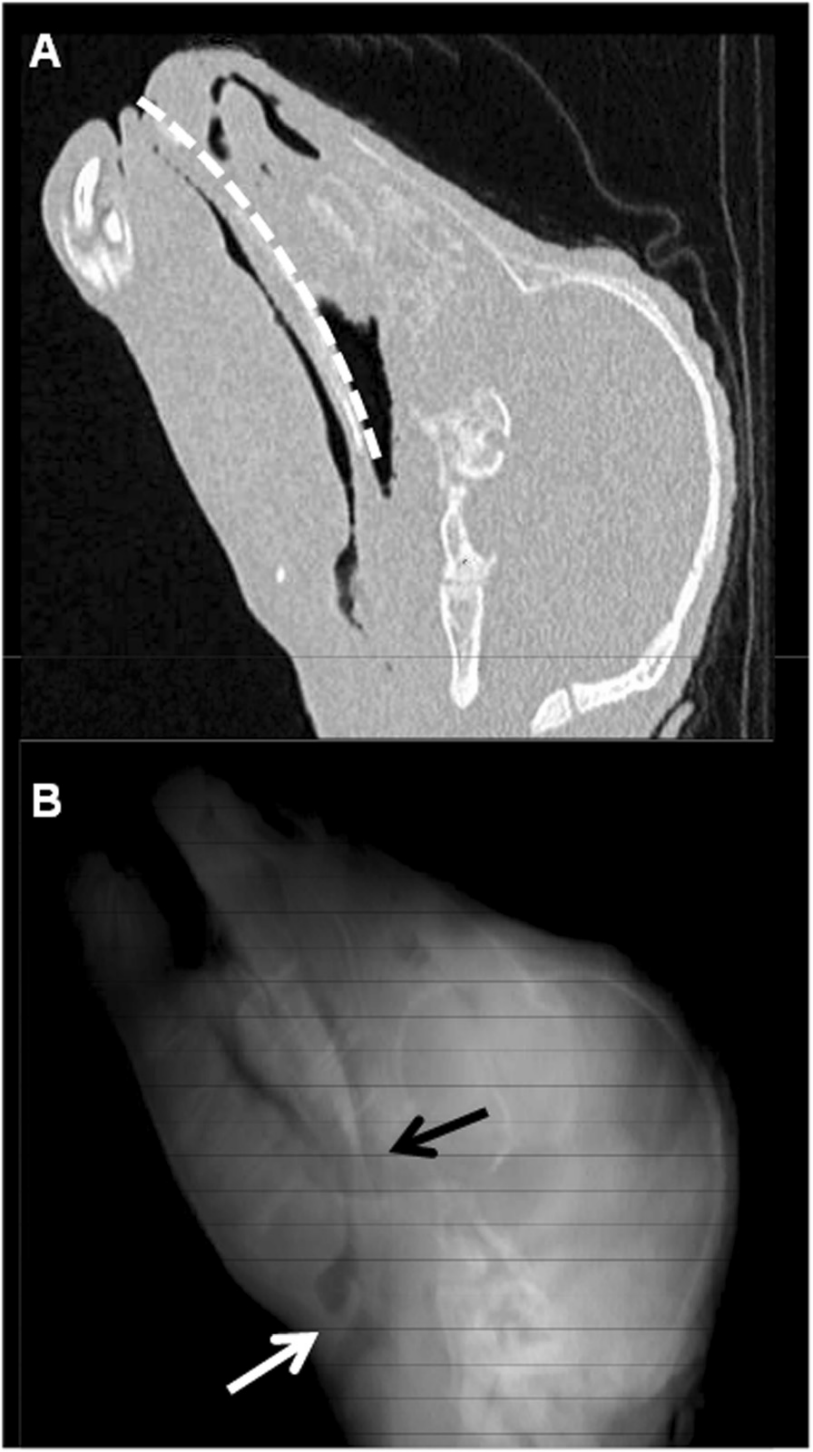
Nasopharyngeal anatomy in the preterm lamb. Computed tomographic images of the snout in a preterm lamb at 129 days gestation. (A) Parasagittal image showing dilatation of the nasal cavity deep within the nasopharynx. Path of nasal CPAP prong indicated by dotted white line (depth 6.5 cm). (B) 3D reconstruction of sagittal CT images; position of CPAP prong tip and epiglottis indicated by black and white arrows, respectively.
